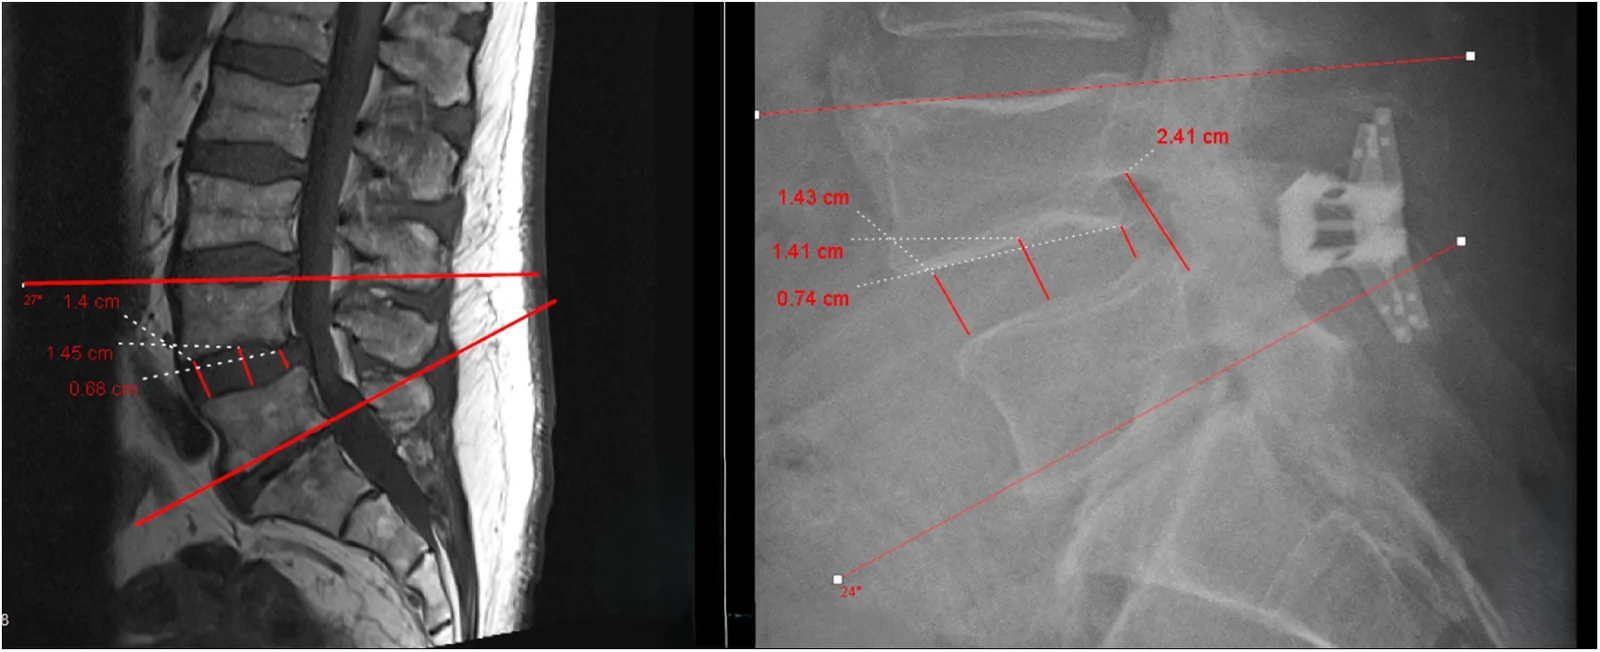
MRI and radiographic example assessment of disc height.
